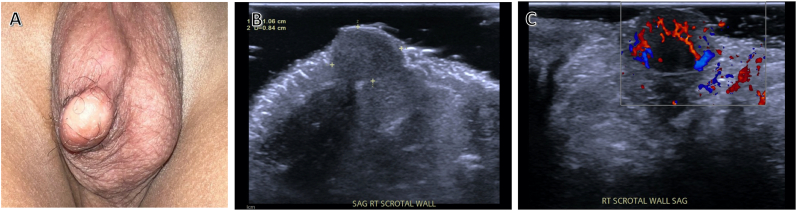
(A) Scrotal lesion on physical examination (B) Scrotal wall ultrasound demonstrating solid lesion (C) Color Doppler image showing internal vascular flow.. (For interpretation of the references to colour in this figure legend, the reader is referred to the Web version of this article.)
